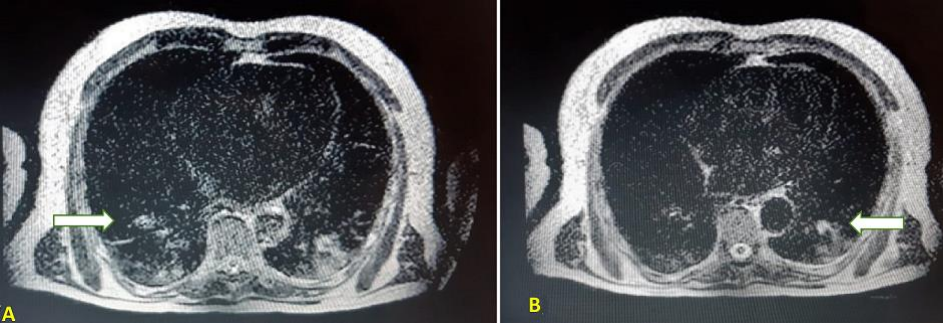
magnetic resonance imaging of the thorax showing multiple pulmonary metastatic lesions; (A) right lobe; (B) left lobe (arrows)
